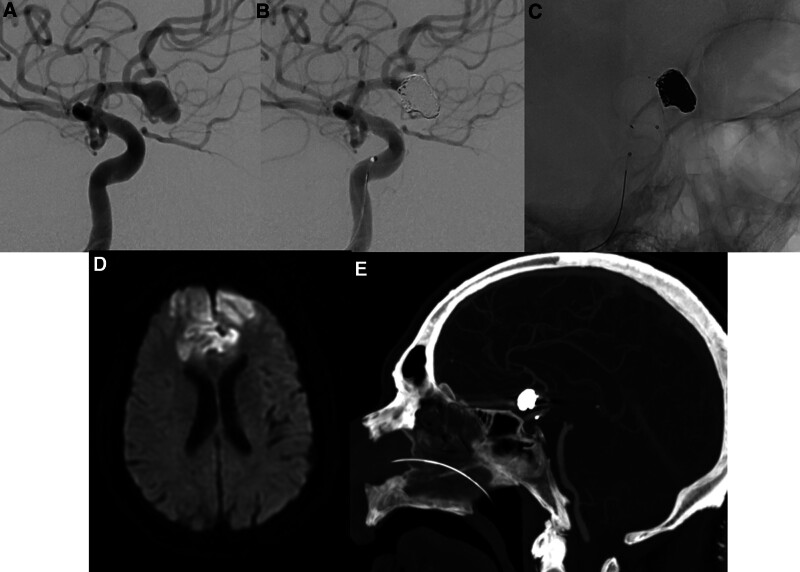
Patient 3 presented with an unruptured ACOM aneurysm (A). pCONUS 2 HPC assisted coiling was performed with complete aneurysm occlusion (MRRC I), as demonstrated on the immediate post angiographic image (B). (C) Unsubtracted image of the deployed stent and coil mass was as shown. Patient was discharged well and readmitted 10 d later for bilateral ACA territory infarcts (D). Of note, bilateral ACAs seen remained patent on the CT angiogram (E). ACA = anterior cerebral artery, ACOM = anterior communicating artery, MRRC = modified Raymond-Roy classification.
